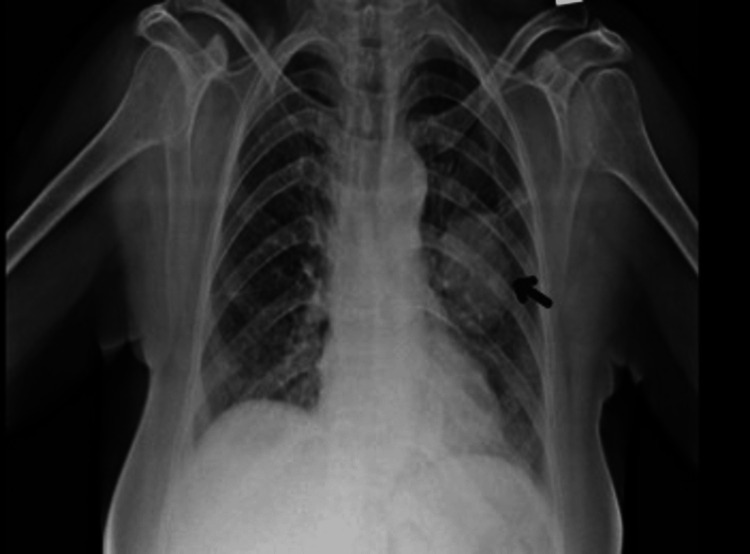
Chest radiograph showing a well-defined mass-like lesion in the left upper zone
